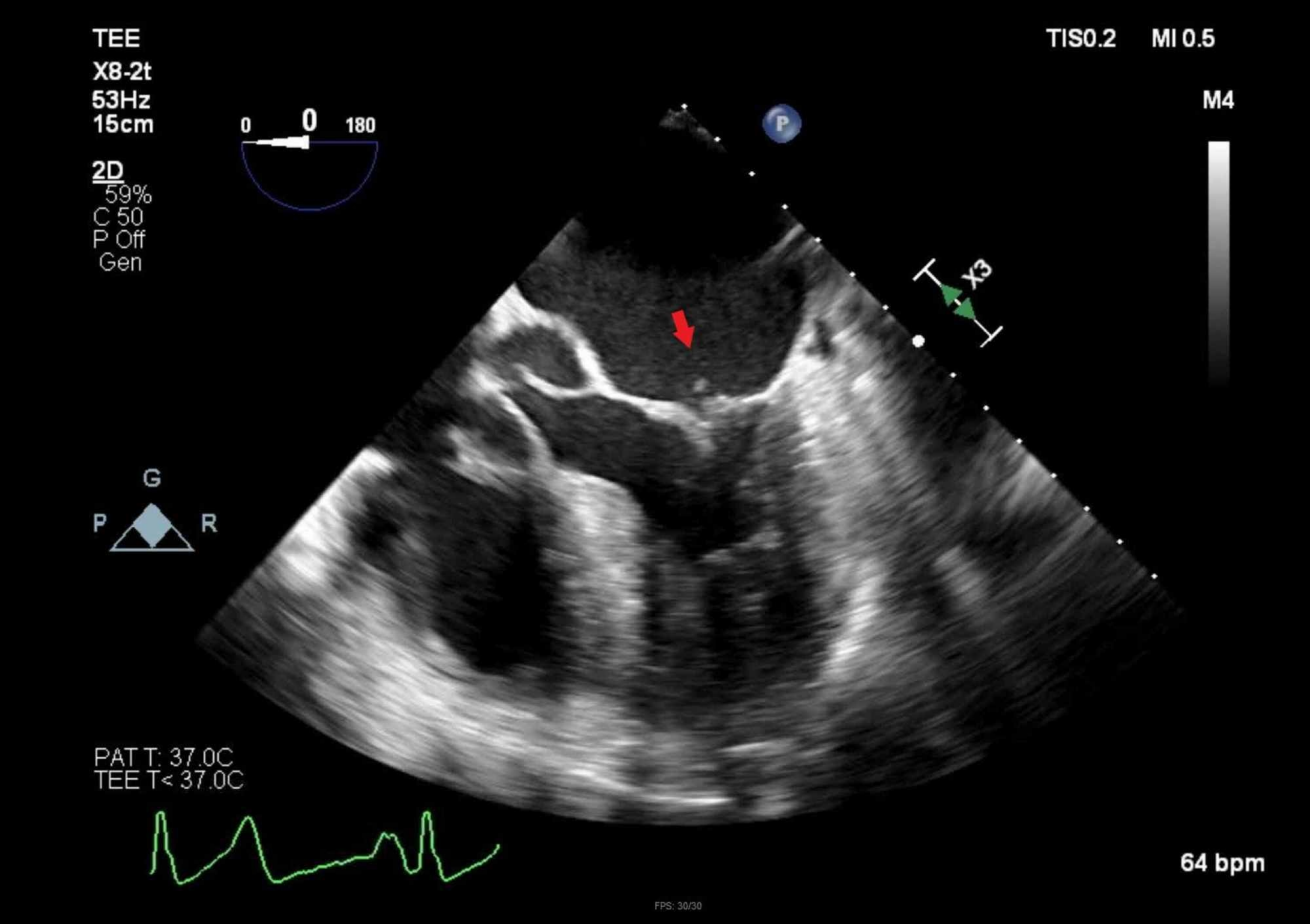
Transesophageal echocardiogram (TEE) revealing the mitral valve with vegetation (arrow)
